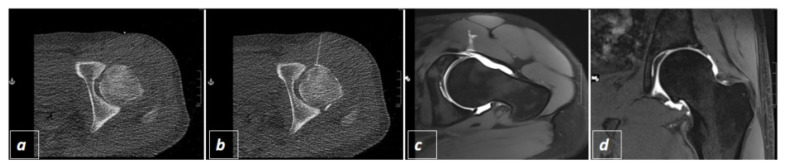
Example of CT-guided hip injection displaying the radio-opaque target as location for the needle insertion (a). Visualization of performed needle path and intraarticular distribution of Iopamidol following application (b). Visualization of intraarticular application of Gadopentetat-Dimeglumin resulting in good joint distension as demonstrated in the following MR-arthrography in transversal (c) and coronal (d) T1 weighted fat-saturated images.
